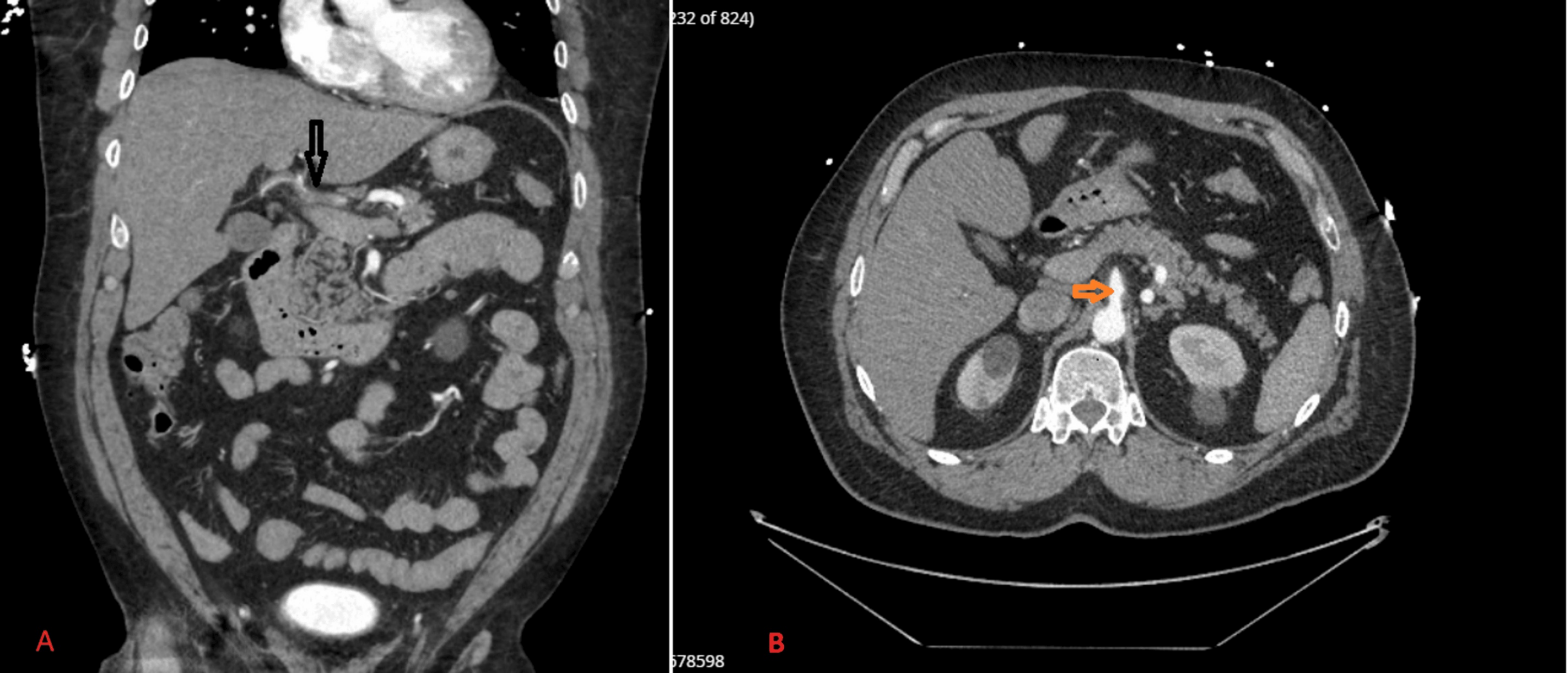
1A. Arterial phase CTA abdomen coronal view demonstrating a common hepatic artery dissection with intraluminal thrombus (black arrow). 1B. Arterial phase CTA abdomen axial view showing a patent celiac artery without extension of dissection (orange arrow). CTA: Computed Tomography Angiography
